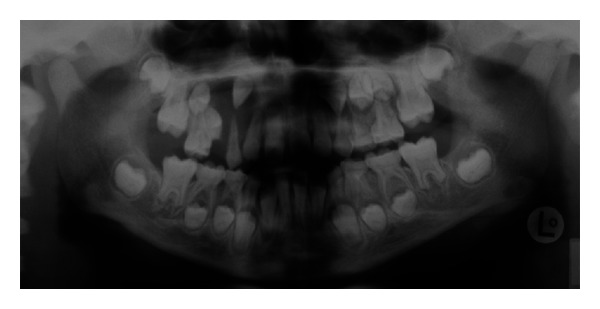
Panoramic radiographic view of the patient at first visit.
